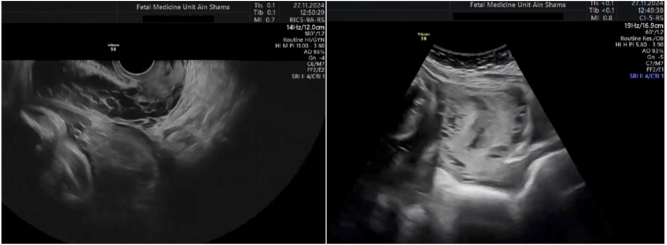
An ultrasound showing a non-gravid uterus, an extrauterine viable fetus.
